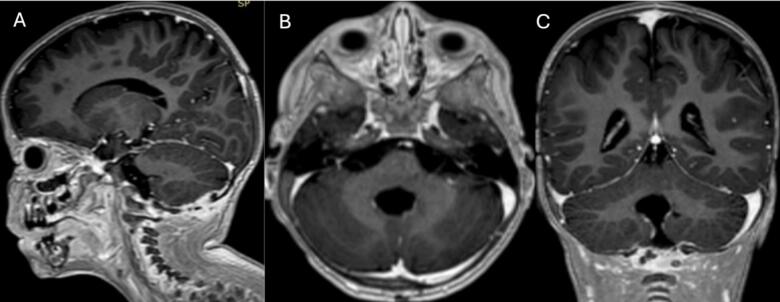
Magnetic resonance imaging of the brain: First imaging after the second craniotomy showing complete resection.
